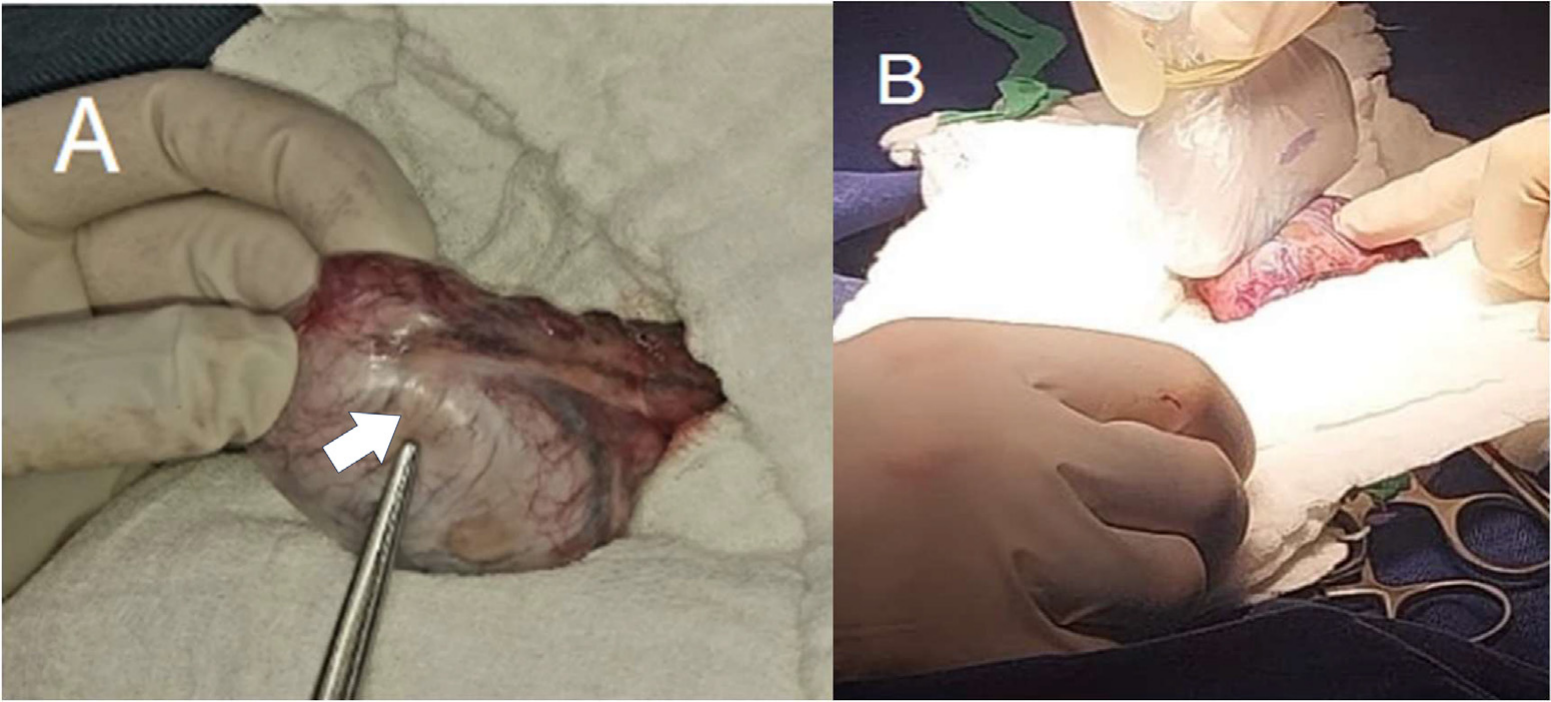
(A) Macroscopic identification of the lesion (Arrow). (B) Intraoperative ultrasound.
